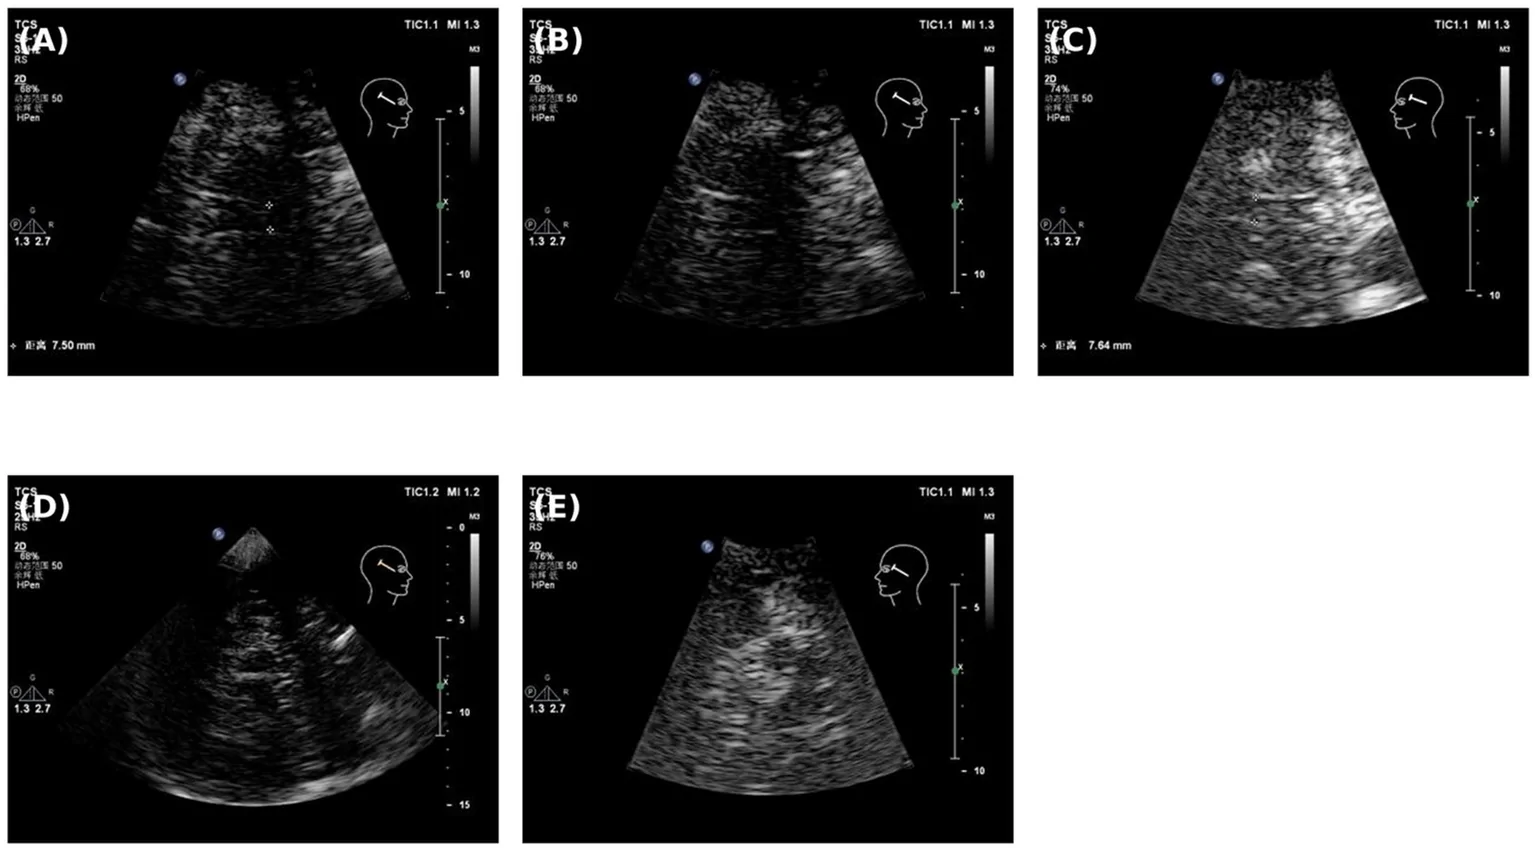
Transcranial sonography of the third ventricle, lentiform nucleus, and brainstem raphe. (A) Third ventricle width measurement. (B) Lentiform nucleus with homogeneous low echogenicity (negative). (C) Lentiform nucleus with patchy hyperechogenicity (positive). (D) Normal brainstem raphe echogenicity (negative). (E) Interrupted brainstem raphe echogenicity (grade 0).
